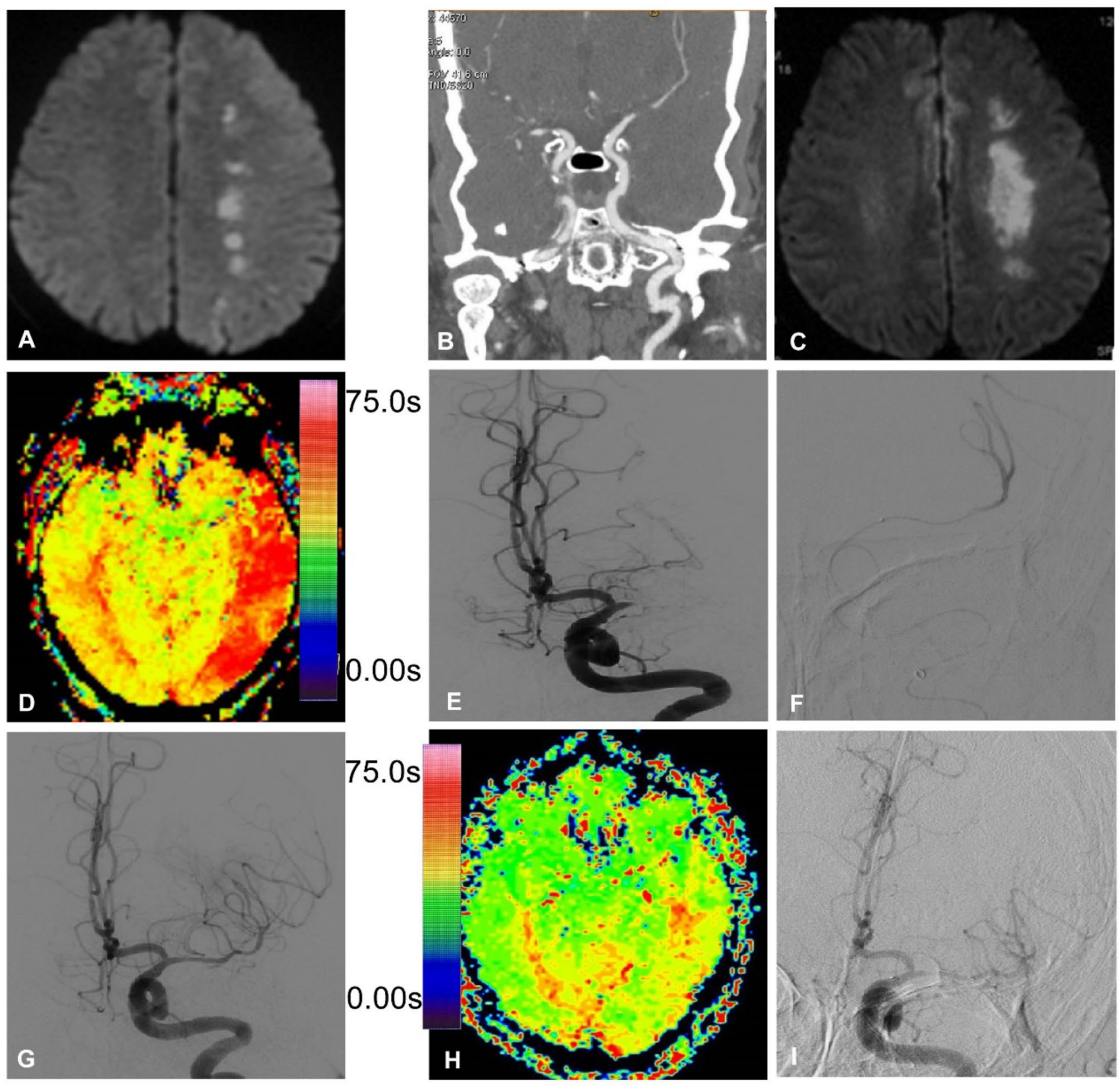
Endovascular recanalization of occluded left middle cerebral artery (MCA) and angiographic follow-up. (A) DWI of magnetic resonance imaging (MRI) immediately after admission revealed bead-like high signal in the left hemisphere. (B) Computed tomography angiography showed occluded M1 segment of the left MCA. (C) Two days after admission, DWI of MRI demonstrated increased area of high signal lesions in the left hemisphere with fusion of the original bead-like lesions. (D) PWI of MRI revealed greater time needed for the blood flow to reach the left hemisphere. (E) Digital subtraction angiography showed occluded M1 segment of the left MCA with no display of the distal arterial branches. (F) A microguidewire was used to explored the occluded M1 segment, and a microcatheter was navigated along the microguidewire through the occluded M1 segment into the M2 segment of the left MCA, and angiography through this microcatheter indicated patency of distal arterial branches. (G) An Enterprise 4.5 mm*22 mm stent was deployed at the occluded segment after balloon angioplasty, with recanalized arterial branches of the left MCA. (H) PWI of MRI demonstrated improved blood flow to the left hemisphere, much better than before recanalization. (I) Digital subtraction angiography six months later revealed patent left MCA with no instent stenosis.
